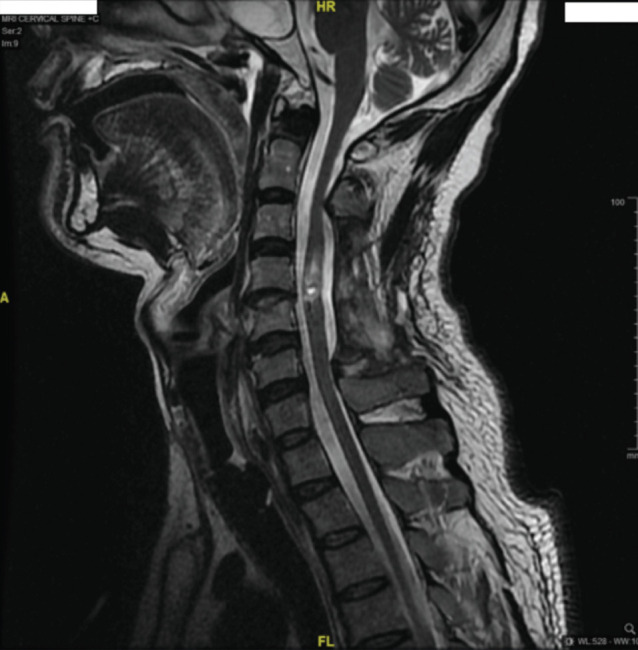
Another MRI of cervical and thoracic spine at day 34 post injury showed an increased T2 weighted signal from C4 to C5 with cord.
